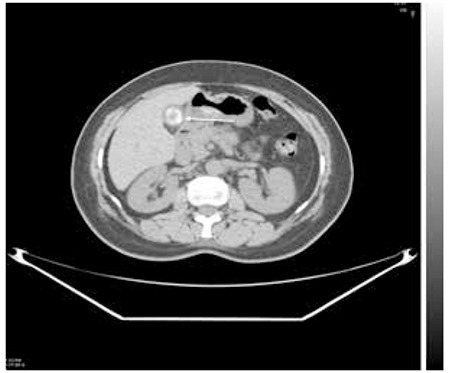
Abdominal Computed Tomography (CT) image with presence of gallstone
